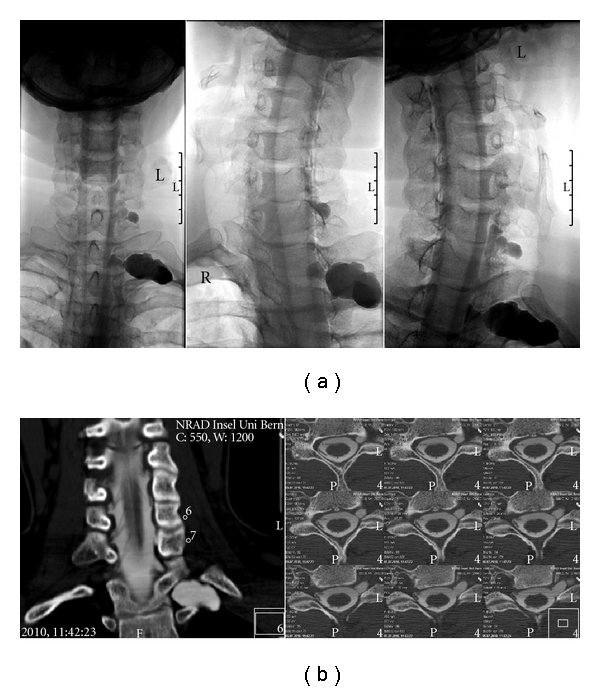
Cervical root avulsion after motorcycle accident. (a) Myelography shows traumatic pseudoceles C7-D1. Rootlets are not discernible. (b) Thin-section (1.25 mm) myelo-CT and reformatted coronal images clearly show complete avulsion of ventral and dorsal rootlets.
